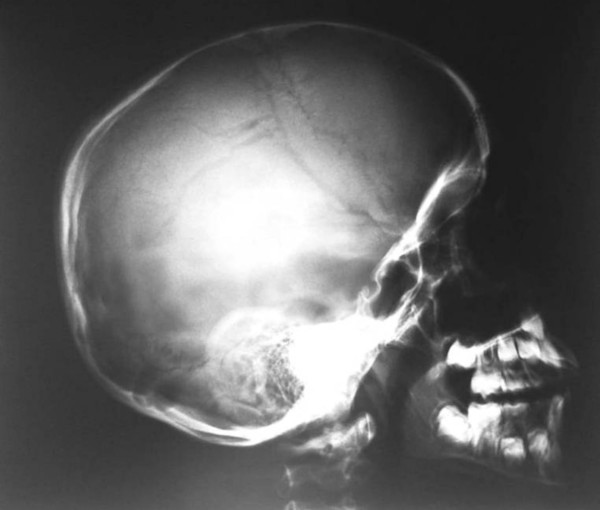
Lateral skull radiograph showed diffuse skull-base sclerosis associated with J-shaped cella turcica and a large sphenoid sinus, multiple patent sutures, mild nasomaxillary/mandibular hypoplasia and odontoid hypoplasia.
